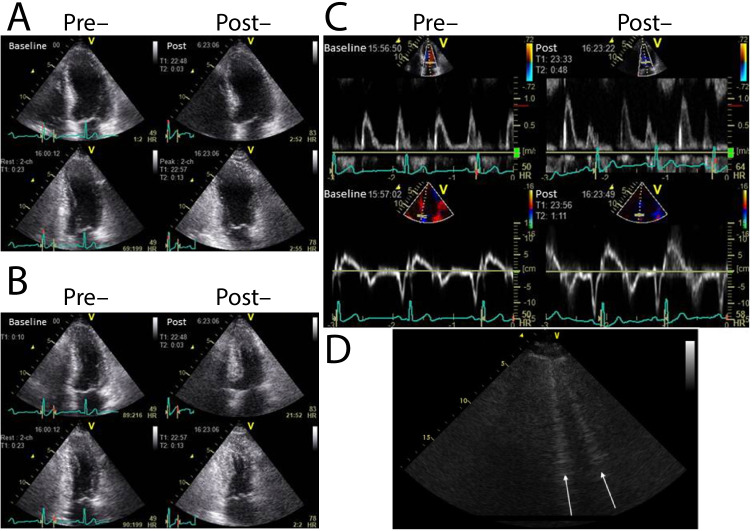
Echocardiography prior to and post exercise can reveal comparatively subtle increases in diastolic dysfunction. Reproduced here is the case of a 75-year-old male with current exertional dyspnoea and a history of percutaneous coronary interventional therapy. Wall motion analysis revealed no exercise-induced wall motion abnormality in either view (A, B). The measured mitral flow pattern and tissue Doppler was consistent with that of delayed relaxation (C) and E/E’ increased from 14 at rest to 16 with exercise [127]. Similarly, hyperechoic b-lines that arise in the lung from transudated fluid (D) that can be detected in HFpEF patients with dyspnoea, with good prognostic power [128]
